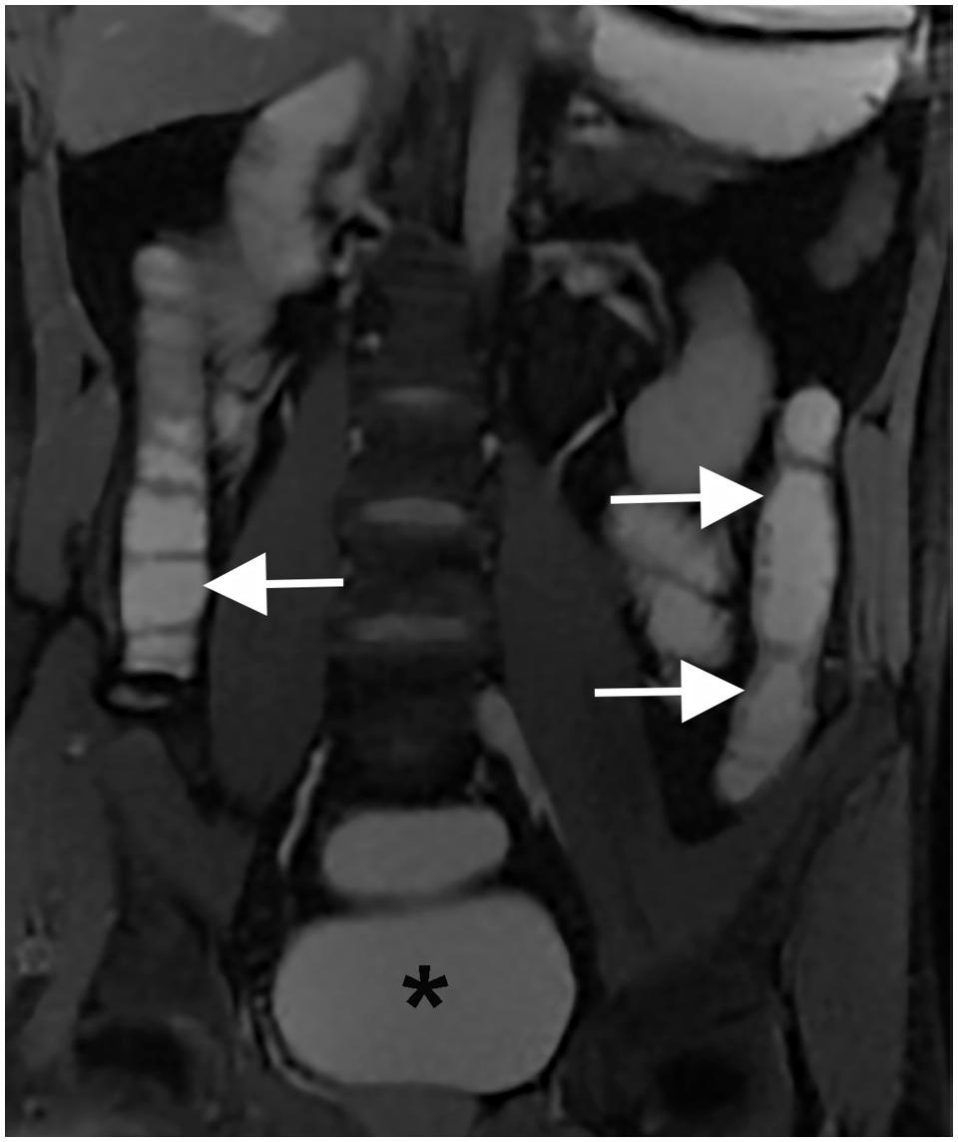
Hydro-MRI in a patient with known Crohn’s disease: coronal T2-weighted image after rectal enema (black asterisk) shows an acceptable distension of ascending and descending colon (white arrows).
